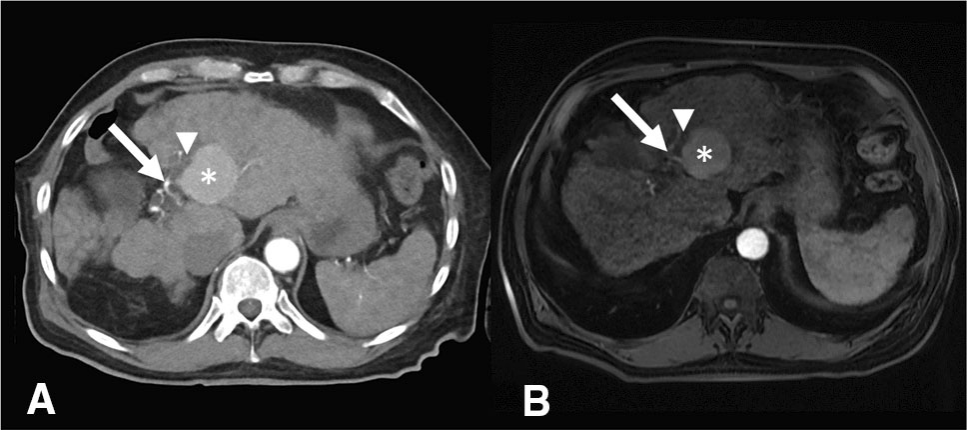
75-years old male patient with Child A liver cirrhosis and a 4.7 × 4.5 × 3.5 cm large central HCC BCLC B involving liver segments IV/VIII (asterisks). A Contrast-enhanced CT in arterial phase. B Contrast-enhanced MRI, T1 vibe 3d fs in arterial phase. Arrows mark close proximity of the tumor to the liver artery; Arrowheads mark close proximity of the tumor to the central bile ducts, resulting in local cholestasis
